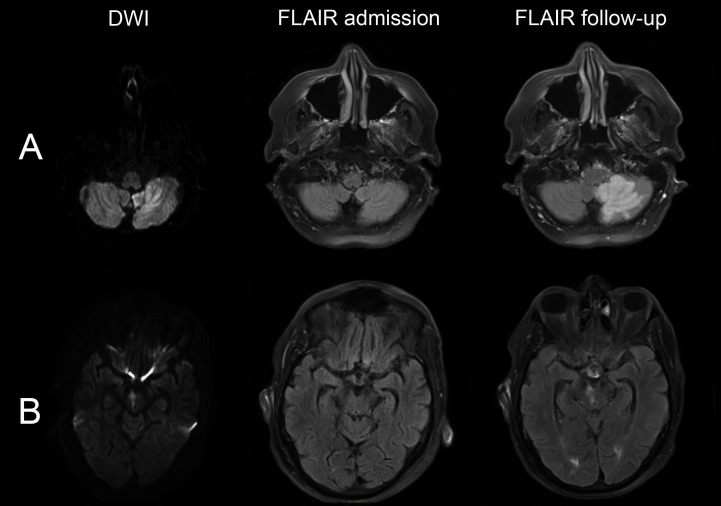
Examples of late FLAIR changes of acute infra-tentorial DWI lesions. The DWI shows a left cerebellar lesion (A) and a right mesencephal lesion (B) 22 h and 16,5 h after symptom onset, respectively. Both have no clear FLAIR visibility on the day of admission but are demarcated on the follow-up examination.
